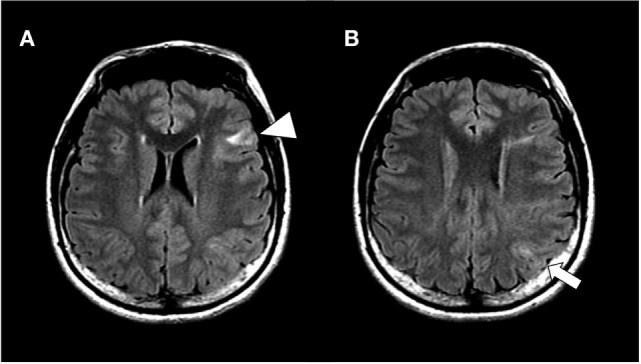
Hippocampal slices of fluid attenuated inversion recovery magnetic resonance imaging at lateral ventricles level (A) and next slice above A (B). The patient showed multiple cortical tubers on bilateral hemispheres, including in the inferior frontal gyrus (arrow head) and inferior parietal lobule (arrow).
